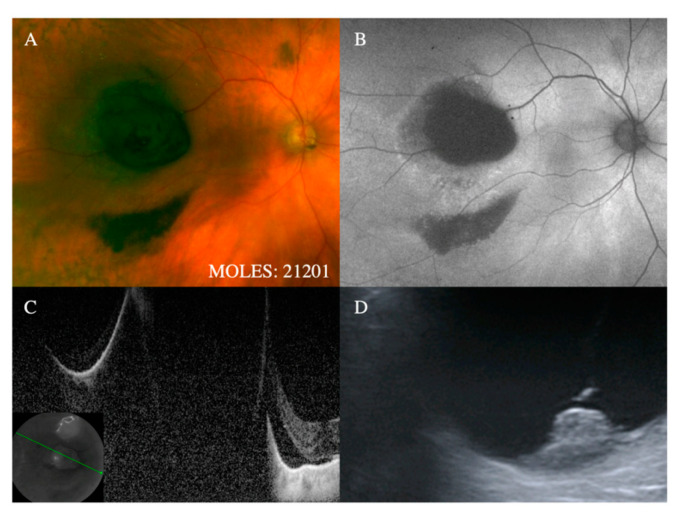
Representative cases demonstrating mushroom shape (i.e., M = 2). (A) Evidence of small nodule formation with associated hemorrhage on color photography and corresponding (B) hypo-autofluorescence with evidence of (C) a nodule on optical coherence tomography (OCT) and (D) confirmed on B-scan ultrasound (M = 2).
